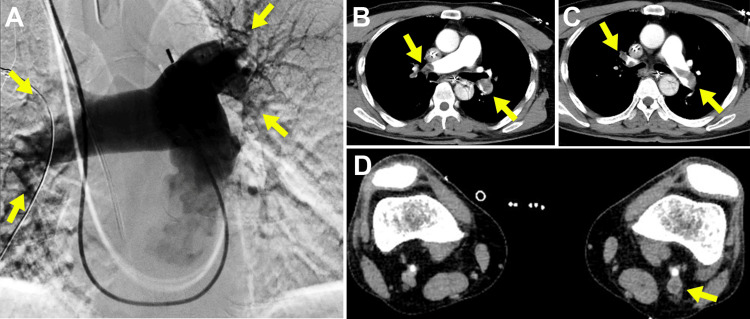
Pulmonary angiography Pulmonary angiography reveals bilateral pulmonary artery occlusion (A). Contrast-enhanced computed tomography reveals pulmonary embolism (B, C) and venous thrombosis in the left popliteal vein (D). Arrows indicate blood vessels occluded by thrombus.
